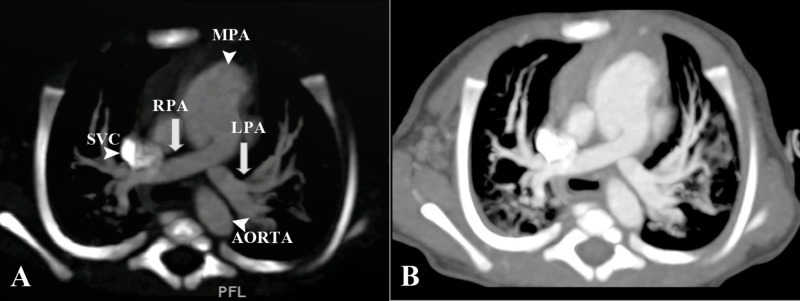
CT cardiac angiography axial sections “Crisscross” pattern of right pulmonary artery (RPA) and left pulmonary artery (LPA) (white arrows). Also labelled are the main pulmonary trunk (MPA) superior vena cava (SVC) and descending aorta (arrowheads).
